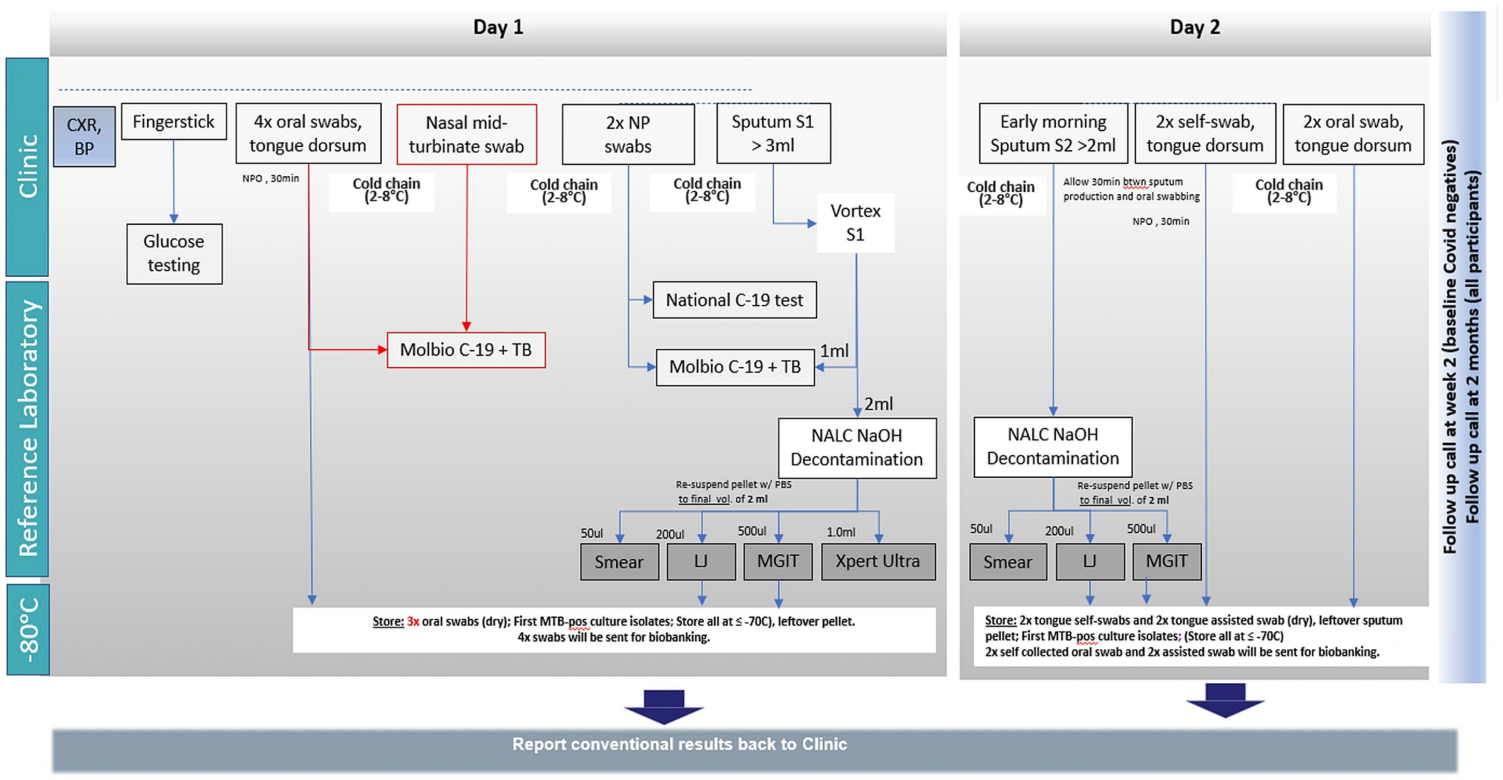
Schedule of assessments for sample type and test. BP, Blood pressure; CXR, Chest x-ray; LJ, Lowenstein-Jensen (solid culture); MGIT, Mycobacteria growth indicator tube (liquid culture); NP, Nasopharyngeal; NPO, Nil per oral; TB, Tuberculosis.
